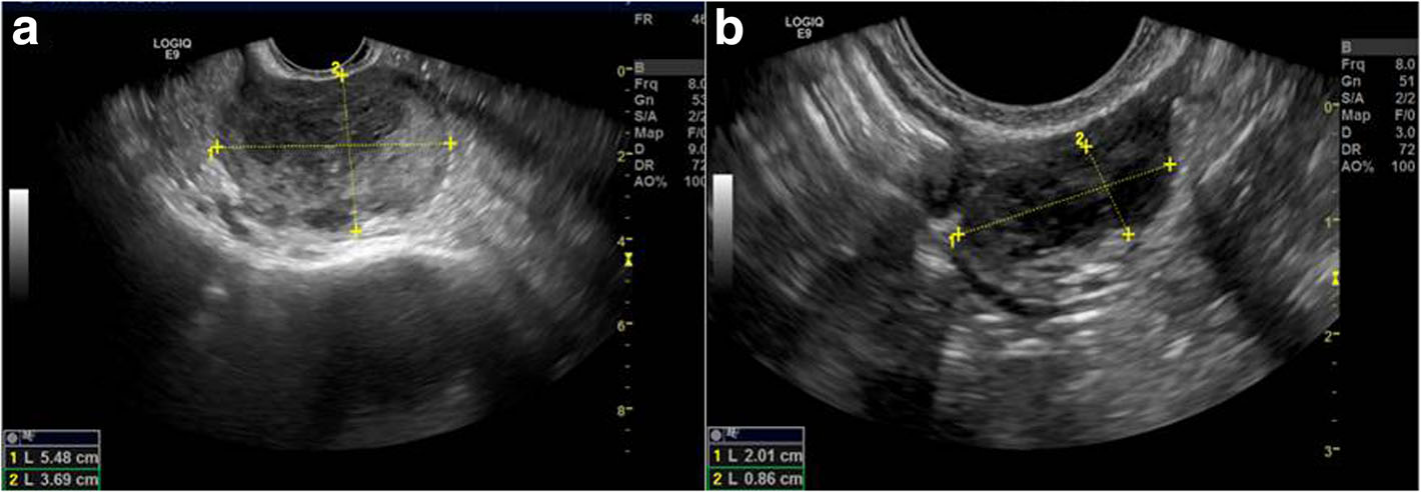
a The solid and hypoechoic pelvic mass detected at trans-vaginal ultrasound. b Ultrasonographic aspect of left ovary
